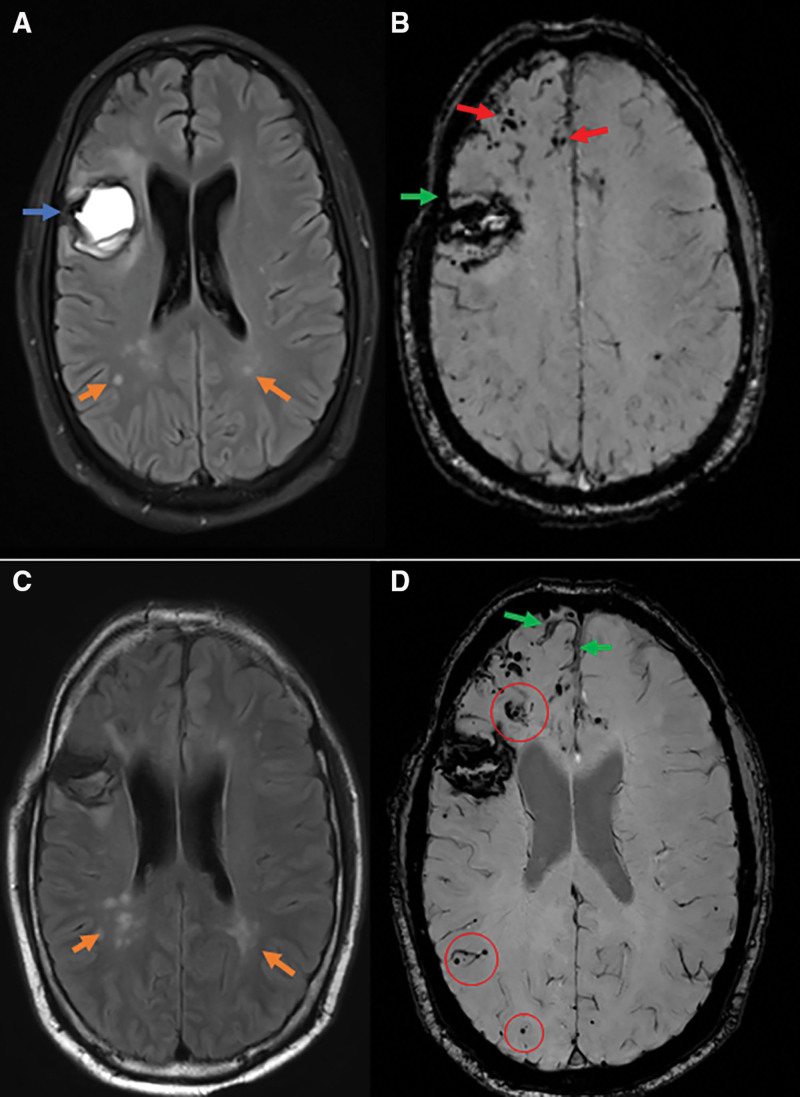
Magnetic resonance imaging (MRI) findings and follow-up of one patient with possible iatrogenic cerebral amyloid angiopathy (CAA). Forty-four-year-old man, with prior history of neurosurgery (1976) for an encephalocele with hydrocephalus, with confirmed use of Lyodura in the right frontal lobe, presenting 44 y later with acute aphasia due to a lobar intracerebral hemorrhage (ICH; A; fluid-attenuated inversion recovery imaging [FLAIR]; blue arrow) that was located in the proximity of the resection cavity. Baseline susceptibility weighted imaging (SWI; B) showed multiple cerebral microbleeds (CMB; red arrows) and cortical superficial siderosis (cSS; green arrow) in the vicinity of the ICH. Four mo after presentation he developed tonic-clonic seizures, no MRI was performed. Follow-up SWI after 11 mo (D) showed progression of cSS (green arrows) and new incident CMBs (red circles) in the right frontal, temporal, and both occipital lobes. Note that due to difference in angulation of slices between baseline and follow-up SWI only the frontal region can be compared on the images shown. Follow-up FLAIR-imaging showed increase of white matter hyperintensities in both parietal lobes (A denotes presentation, and C denotes follow-up; orange arrows).
